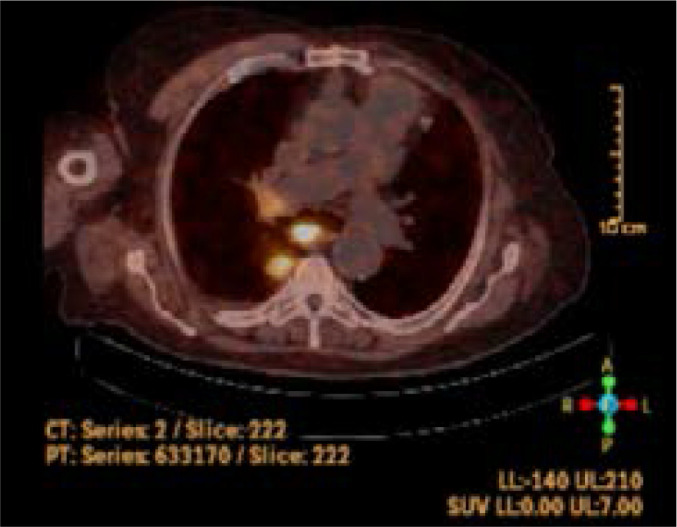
Ga-68 DOTATATE PET/CT scan image of the lesion, axial view
